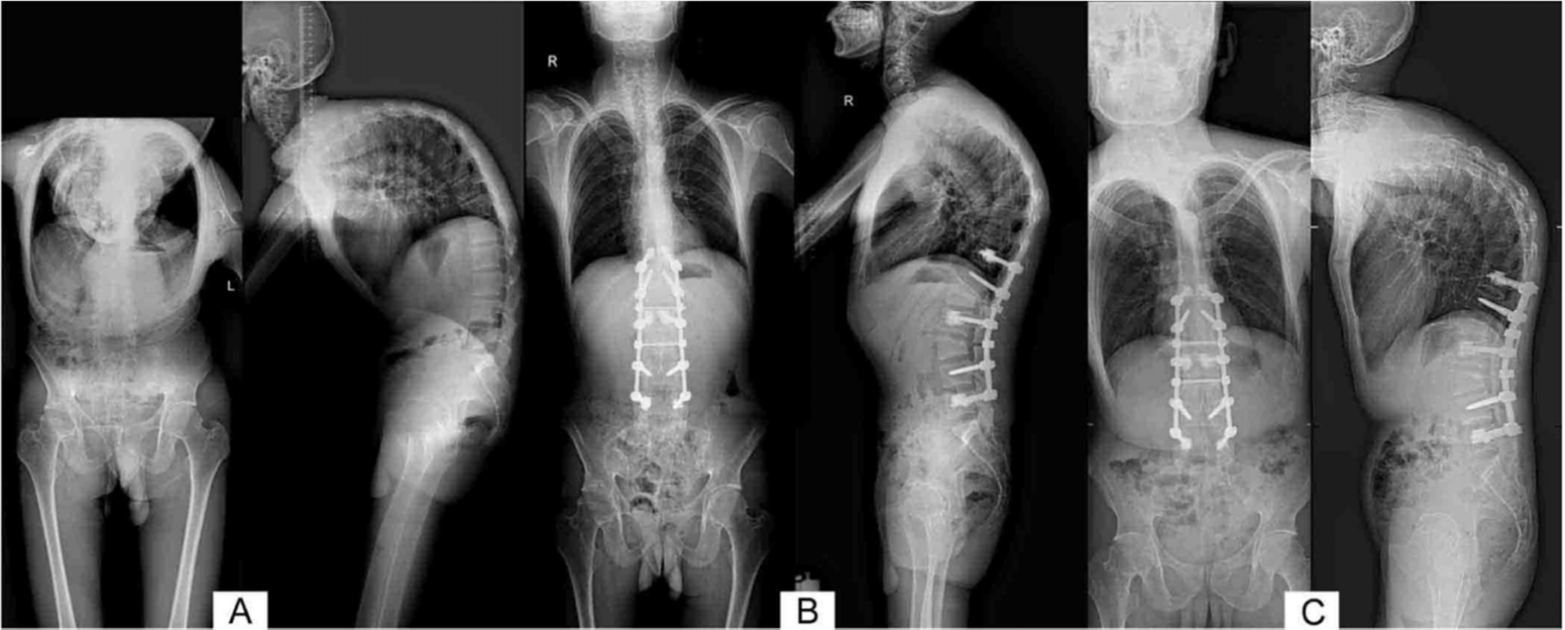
X-ray images. (A) before surgery; (B) immediately after surgery; and (C) at the final follow-up at 52 months in a 31-year-old man with a 4-year history of lower back pain and progressive kyphosis. AS and kyphosis with severe osteoporosis were diagnosed (T-score=–3.0). The patient underwent a partial osteotomy of the key vertebrae without intervertebral fusion. PMMA-augmented CICPSs were used at the ends of the internal fixation instrument, and good spinal correction was obtained.
